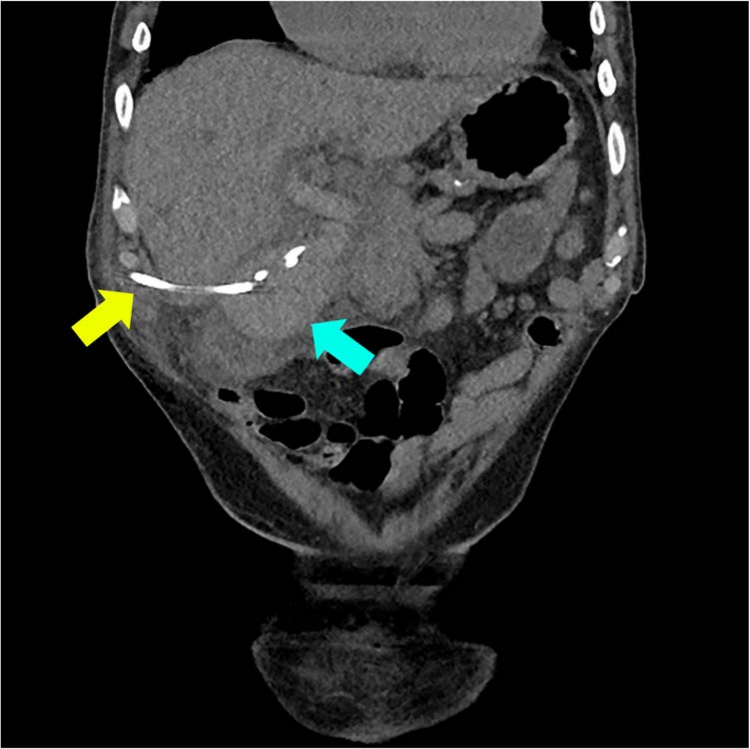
Coronal CT reconstruction showing high density material within the lumen of the gallbladder suggestive of haemorrhage (marked with blue arrow) and cholecystostomy tube (marked with yellow arrow).
